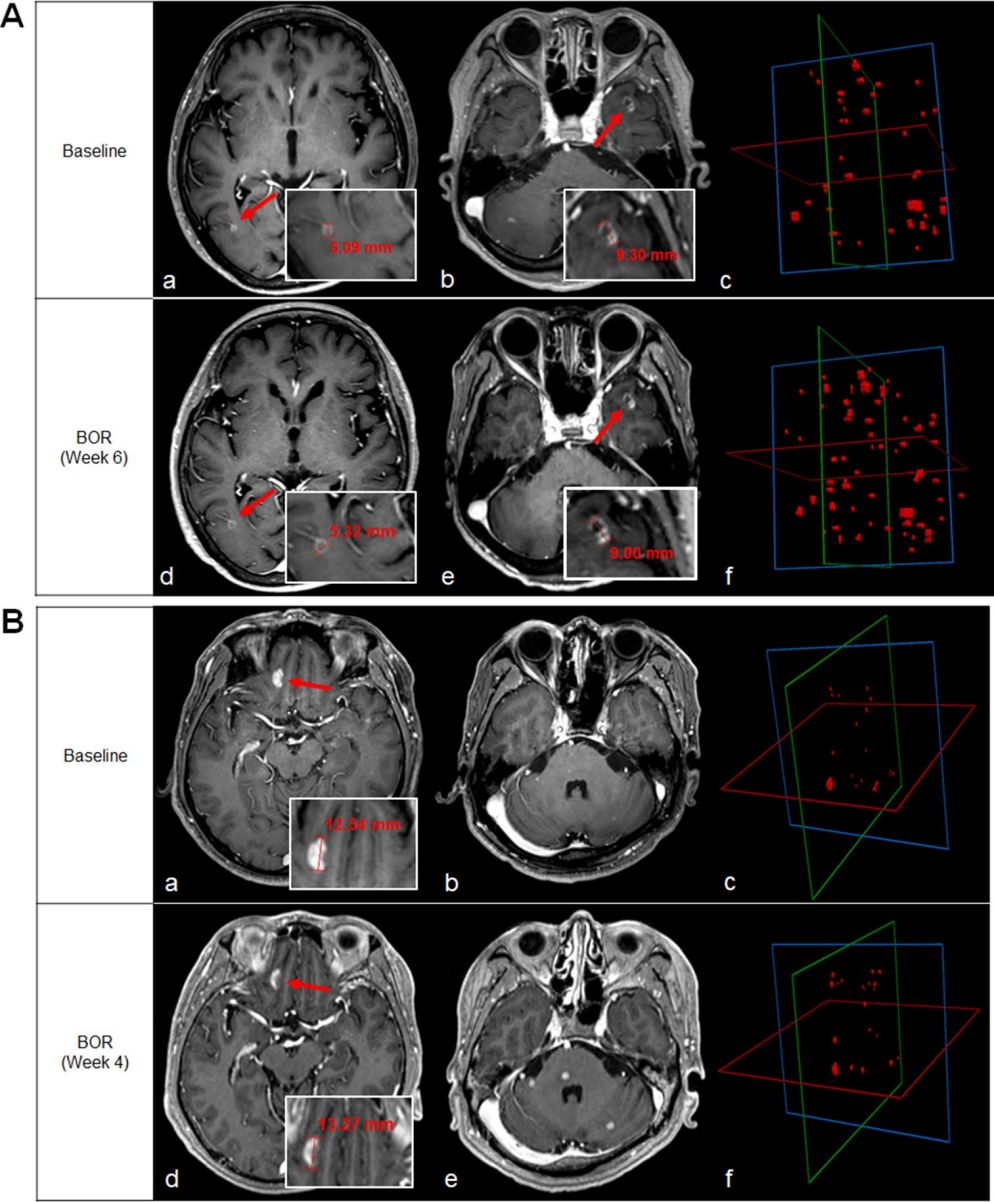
Representative Cases of 3D Response Utility in Predicting Overall Survival A. Baseline scan (a, b) shows two target lesions of 5 and 9 mm. There were also other multiple lesions of less than 5 mm that are not shown in the image. On the MRI at best overall response (BOR) date (d, e), the previous target lesions show no significant change in size, and other multiple enhancing lesions measuring 1–2 mm have appeared (not shown in the image). Volumetric analysis of all segmented measurable and non-measurable enhancing lesions at baseline and the BOR date is shown in (c) and (f). On the baseline date (c), the total volume was 1459.1 mm^3^, and the total number of lesions was 35. On the BOR date (f), the total volume increased by 81.2% to 2643.4 mm^3^, and the measured number of lesions was 60. The patient died about 10 months after the baseline date B. Baseline scan (a, b) shows a target lesion in the right frontal lobe with size of 12 mm and other multiple lesions with size of less than 5 mm. In the MRI at BOR date (d, e), previous target lesion and other multiple non-measurable enhancing lesions shows unequivocal size increase. Volumetric analysis of all segmented measurable and non-measurable enhancing lesions at baseline and the BOR date is shown in (c) and (f). On the baseline date (c), the total volume was 778.5mm^3^ and the lesion number 16. On the BOR date (f), the total volume increased by 70.8% to 1329.4mm^3^, and the measured number of lesions was 21. The patient died about 14 months after the baseline date
